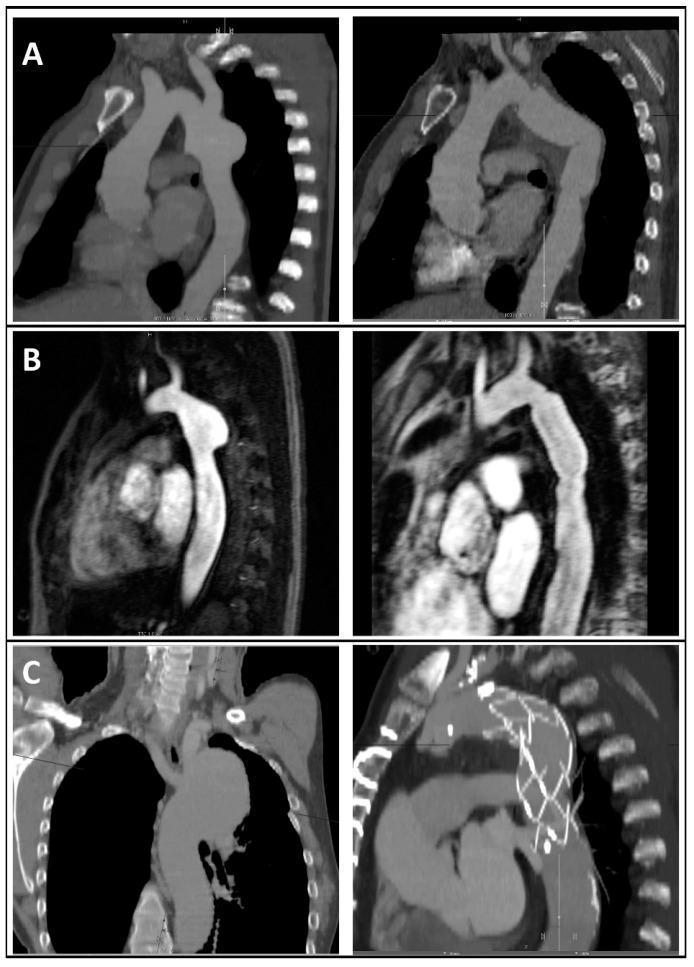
CT angiographic (A,C) or MR angiographic (C) reconstructions of different aneurysm morphologies after coarctation repair (left) and postoperative images after reoperation (right) showing descending aorta replacement and subclavian artery reinsertion in the prosthesis (A), isolated descending aorta replacement (B), or frozen elephant trunk implantation (C).
